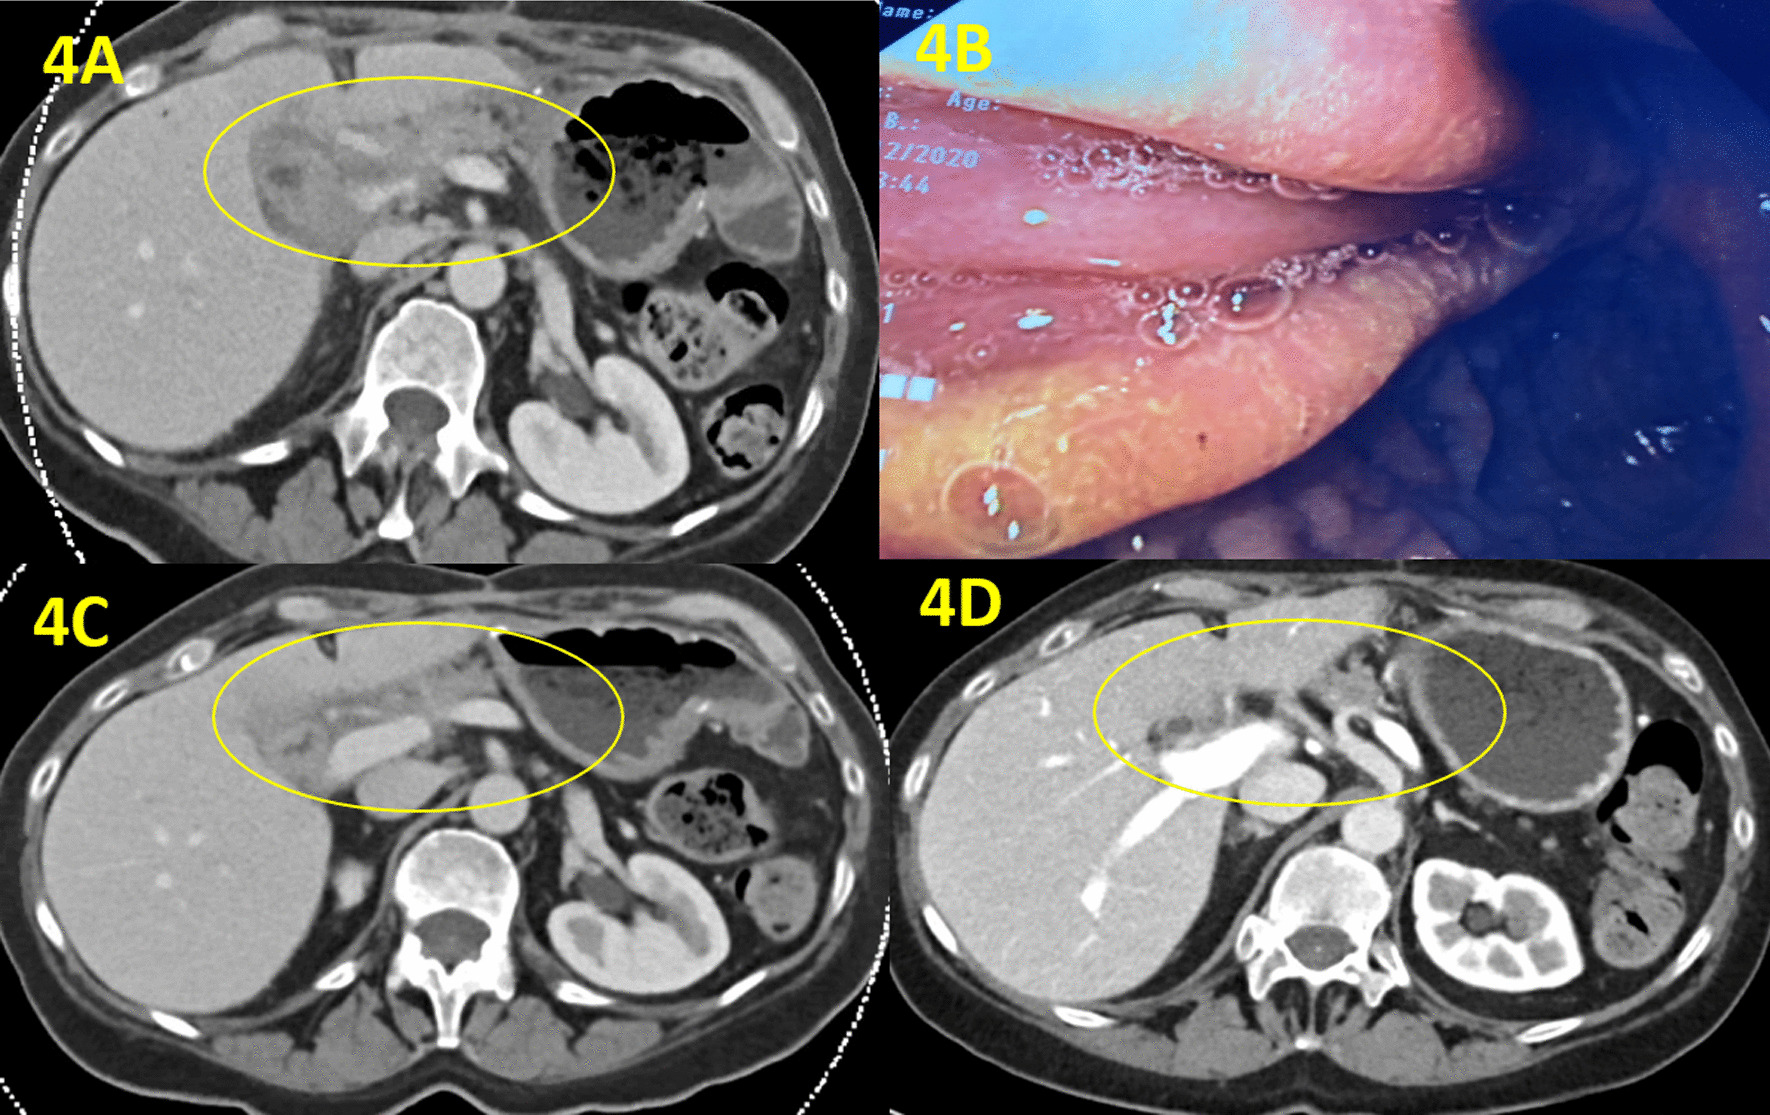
Postoperatively, CT scan revealed inflammatory changes at the anastomosis site with severe duodenal thickening, representing recurrent IgG4-RD (A). Upper endoscopy showed very subtle perianastomotic erosions and erythema (B). Two weeks after prednisone therapy, follow-up CT showed marked improvement of the perianastomotic changes (C). Another follow-up CT at 3 months after starting mycophenolate mofetil therapy revealed no recurrent disease (D)
